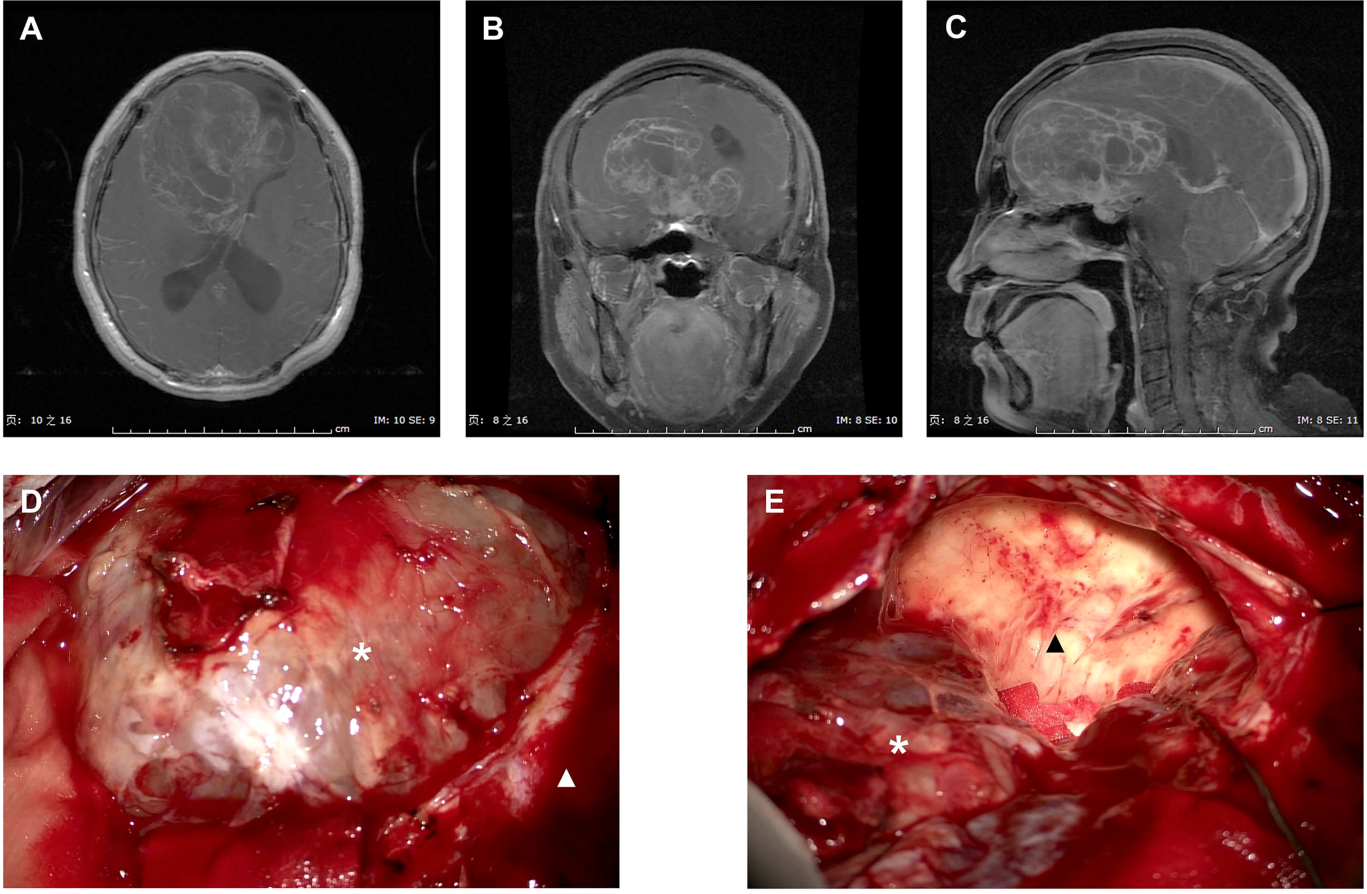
Intraoperative images for tumor resection of a recurrent ISFT. (A–C) Preoperative axial, coronal, and sagittal T1-Gd MRI scans of a huge recurrent ISFT tumor mainly located in the right frontal skull base, showing a marked and heterogeneous enhancement. (D, E) Intraoperative images revealed a red solid tumor mass (black arrow), which was not clearly distinguishable from the normal brain tissue during tumor resection (white arrow). Finally, a subtotal resection was performed due to the tumor invasion of skull base bone and sellar construction with a serious intraoperative bleeding (about 1,000 ml).
